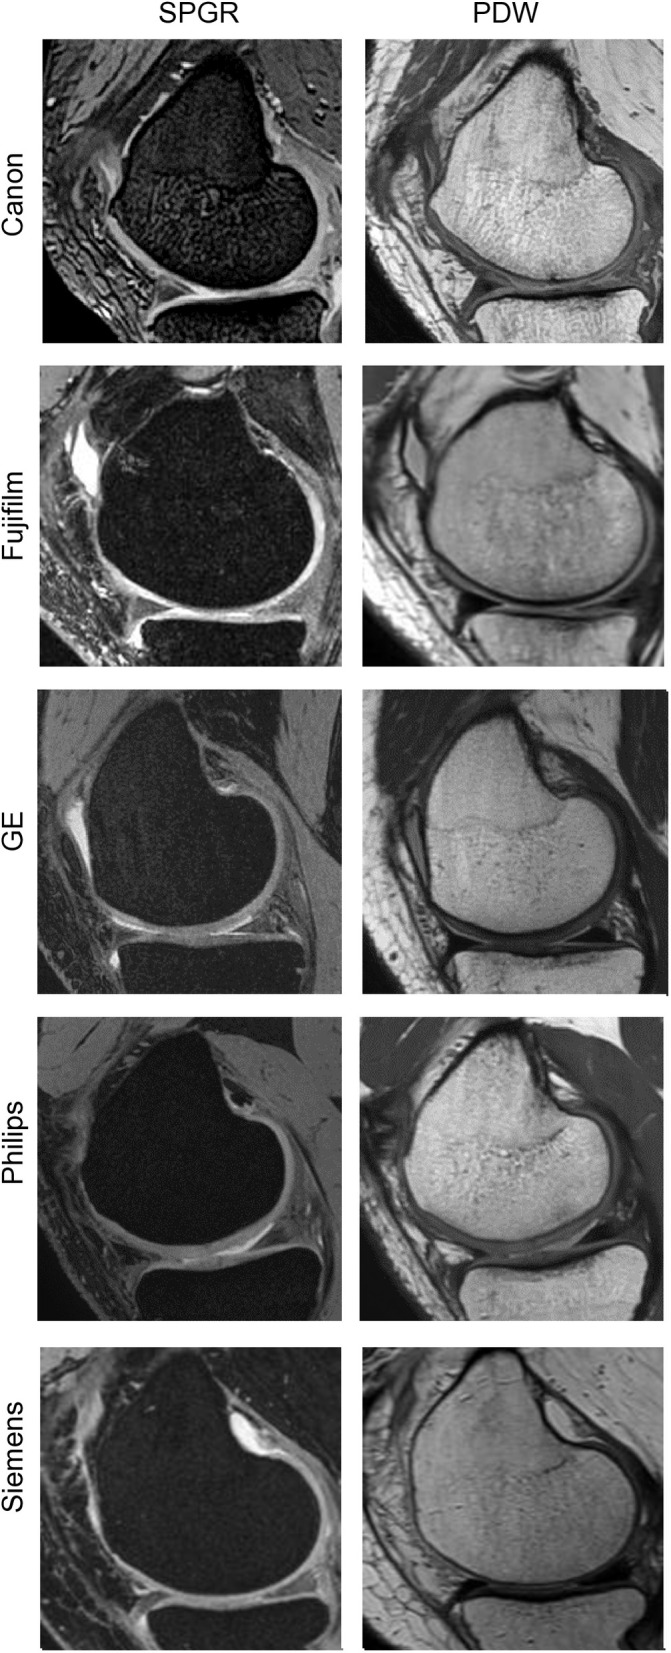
Sagittal MR images of the knee taken with MRI machines from five different companies. Representative examples were selected from each company.
